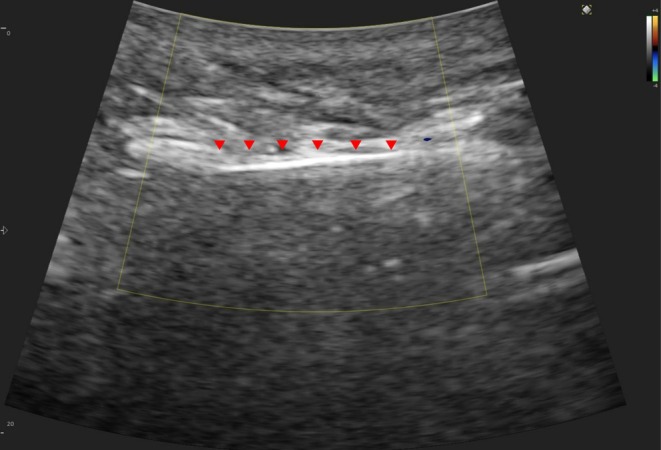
In‐plane mode ultrasound image. The red arrowhead indicates the cannula.
